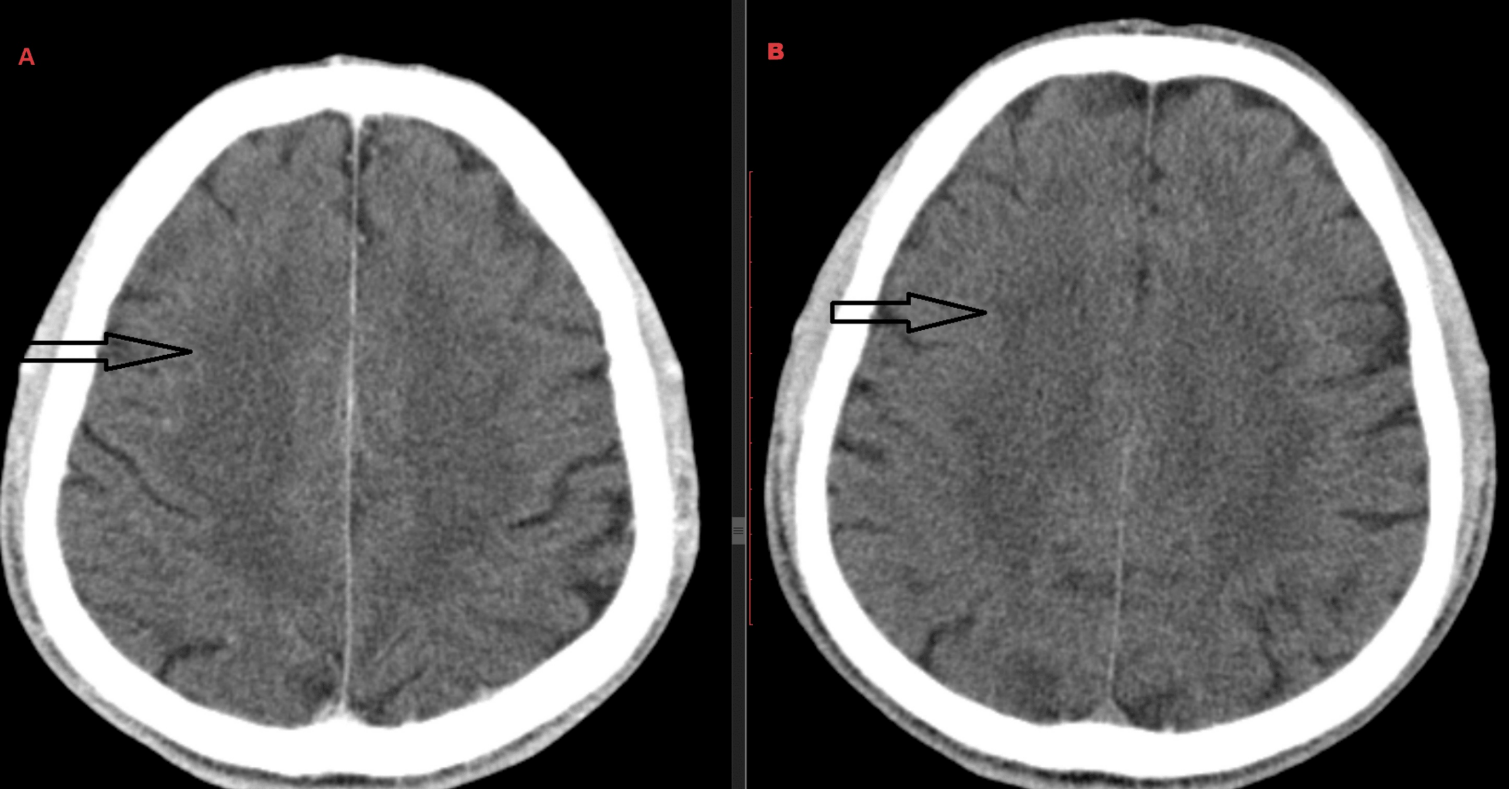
Initial CT brain plain (December 2023) shows no abnormality.
